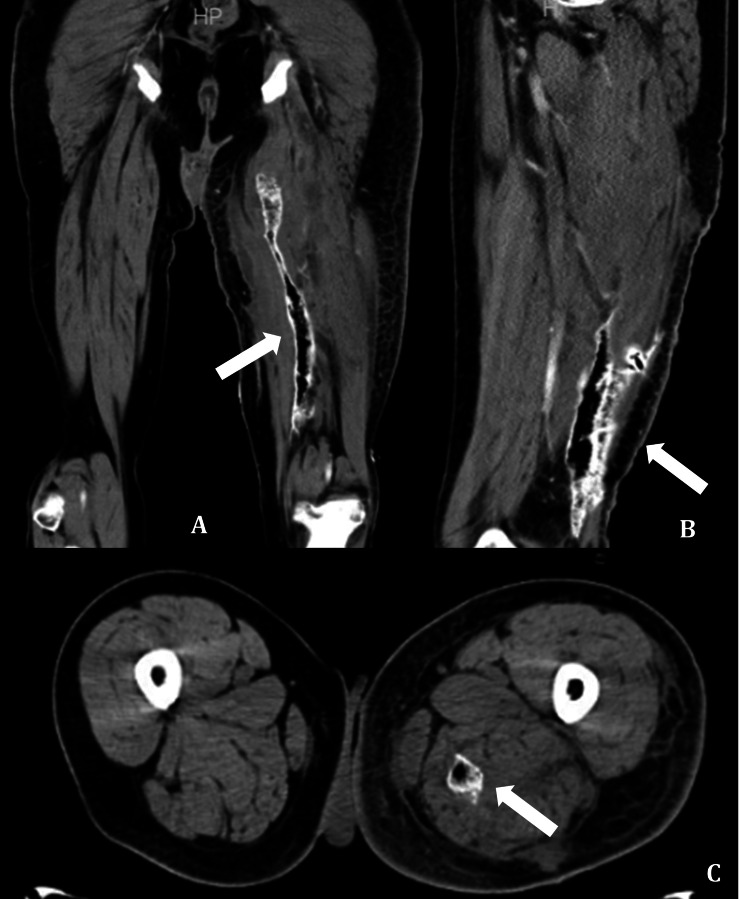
Magnetic resonance imaging of the thighs (T2W post-contrast), depicting an extensive area of necrotizing fasciitis on the posteromedial aspect of the left thigh (arrow). A. coronal view, B. sagittal view, and C. transverse view.
